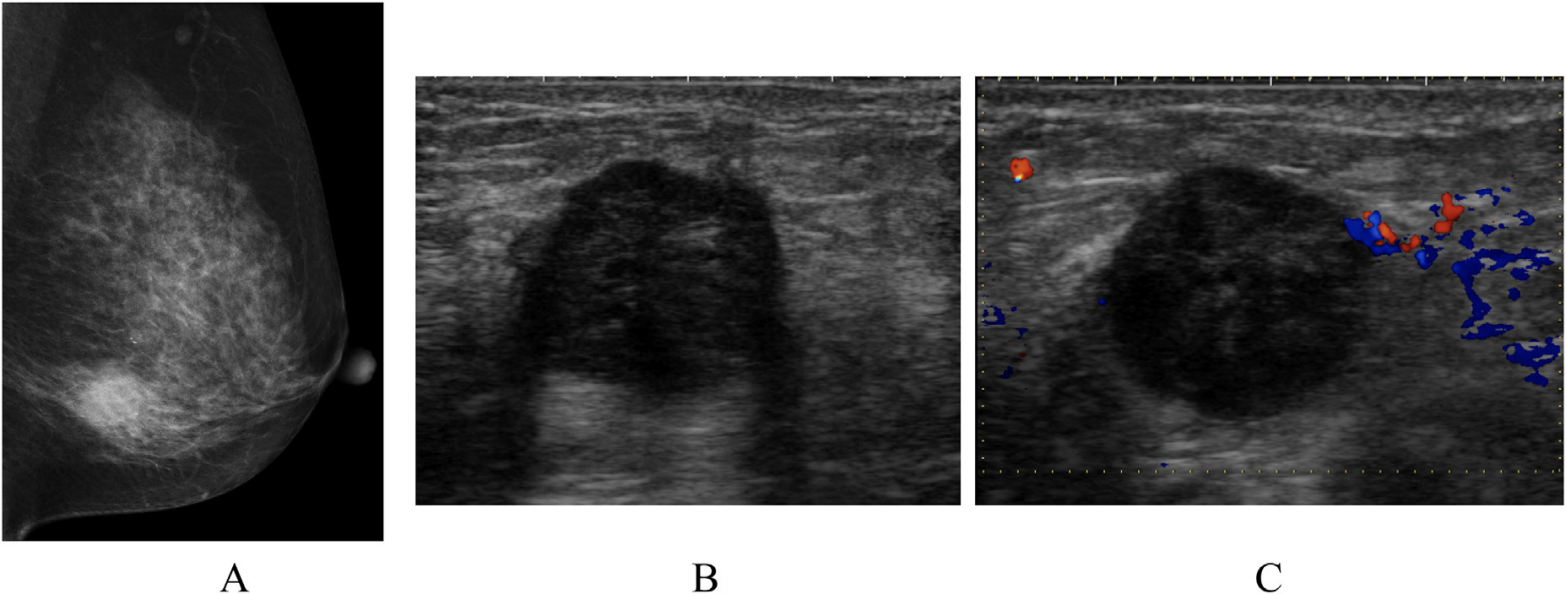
Mammography and ultrasonography. (A) Mammography showed a mass in the lower part of the left breast. (B) Ultrasonography showed a well-circumscribed oval mass with predominantly low internal echoes and enhanced posterior echoes. (C) Doppler ultrasonography showed blood flow just around the tumor.
